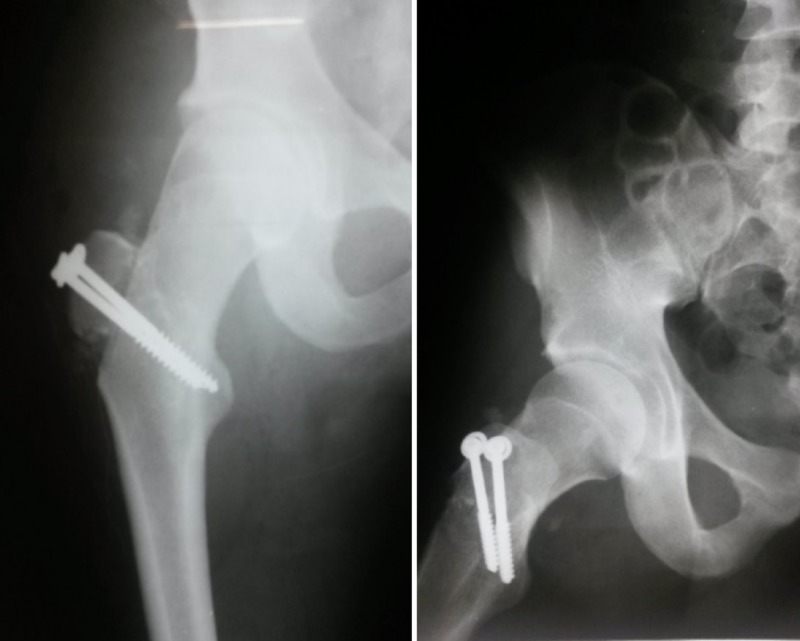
Image of a radiograph after surgery, showing hip reduction and the fixation of the greater trochanter
